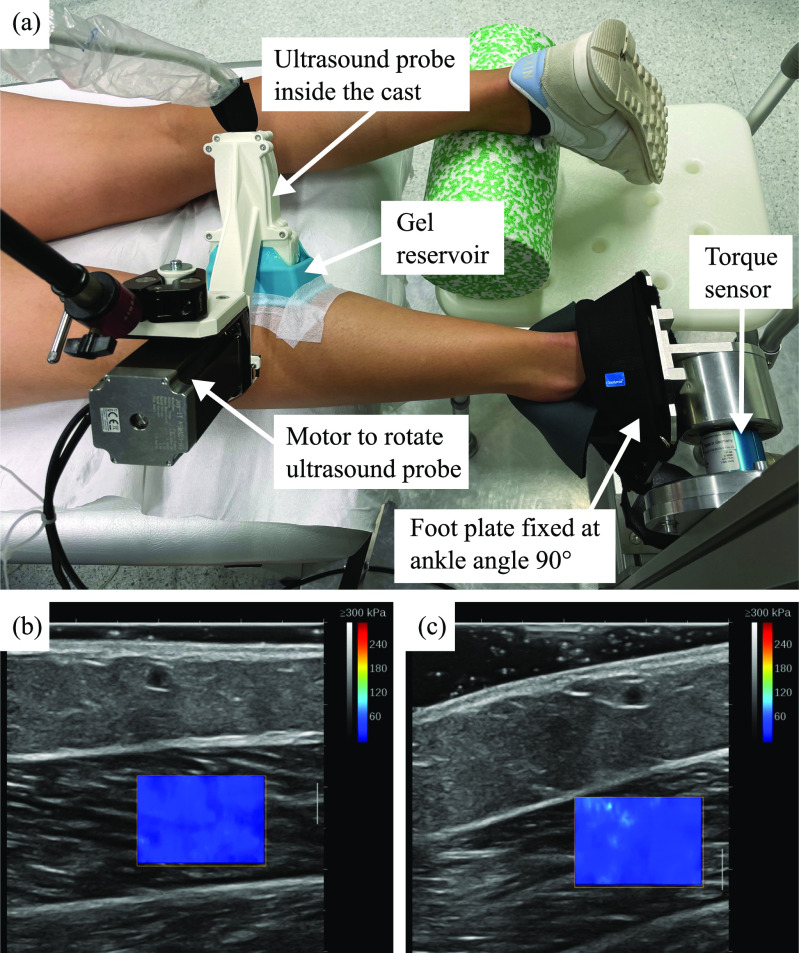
(a) Experimental setup showing a participant during the *automatic aligned* condition. Exemplary ultrasound image during the (b) *not aligned* and (c) *manual aligned* or *automatic aligned* conditions.
